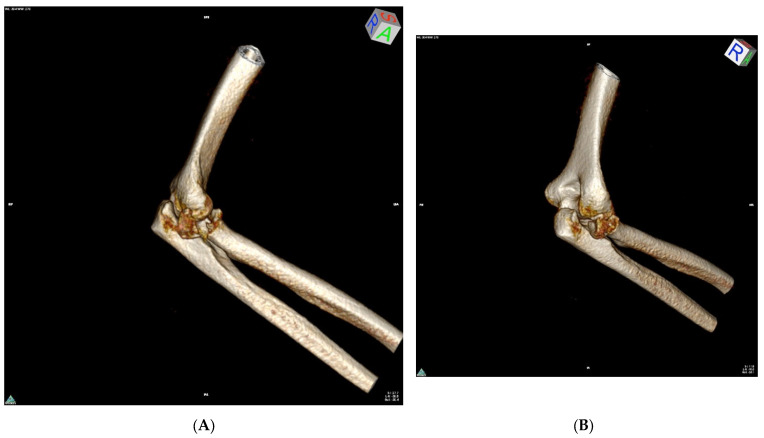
Example of a CT scan (three-dimensional volume reconstruction) showing a Mason type III radial head fracture with significant fracture displacement—P.O. 35y (A,B).
